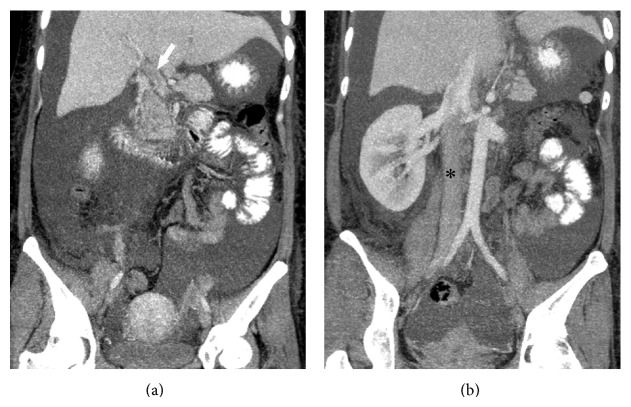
The reformatted coronal CT image showing (a) thrombosis of the main portal vein (arrow), and (b) a long segmental thrombus in the inferior vena cava (∗).
